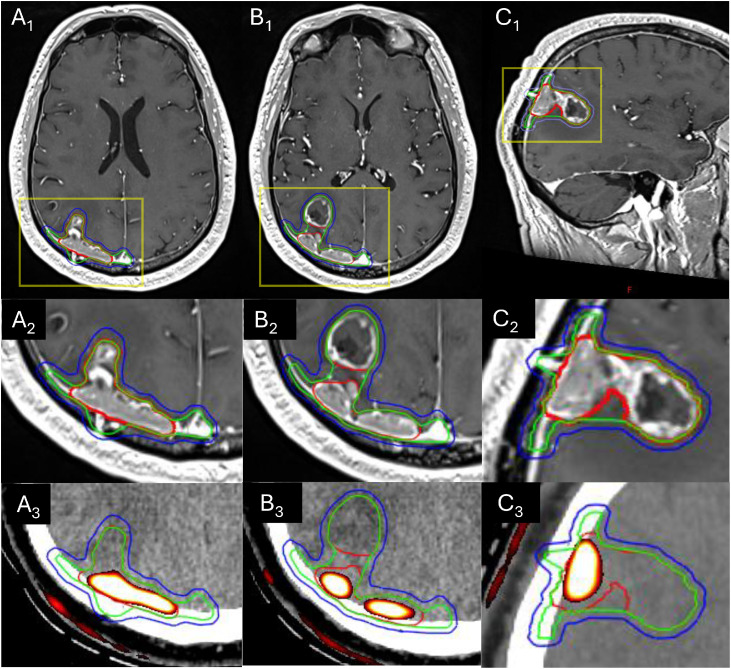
Preoperative reirradiation target volumes for the right parietal lesion with a magnetic resonance imaging (MRI) scan T1c+ and an ¹⁸F-fluciclovine positron emission tomography (PET) scan fusion. Gross tumor volume (GTV, red) encompasses the prior laser interstitial thermal therapy (LITT) tract and operative changes, contrast-enhancing regions, nodular dural disease on an MRI scan, and hypermetabolic regions on an ¹⁸F-fluciclovine PET scan. Clinical target volume (CTV, green) represents an anatomically tailored 3 to 5 mm expansion along the meninges with inclusion of the superior sagittal sinus. Planning target volume (PTV, blue) represents a 2 mm expansion from the CTV. Yellow boxes in A_1_, B_1_, and C_1_ denote magnified views in the middle and lower rows. (A_1_) Full axial MRI scan T1c+ fusion at the level of the burr hole and LITT tract, with a corresponding magnified MRI scan T1c+ view in (A_2_) and planning computed tomography (CT)/PET scan overlay in (A_3_). (B_1_) Full axial MRI scan T1c+ fusion at a more inferior level of the lesion, where the cavitary parenchymal component is more distinct from the nodular dural disease; corresponding magnified views of T1c+ and DSC perfusion overlays are shown in (B_2_) and (B_3_), respectively. (C_1_) Sagittal MRI scan T1c+ at the level of the burr hole and LITT tract, with enhancing parenchymal and nodular dural disease encompassed within the target volumes; corresponding magnified views of T1c+ and DSC perfusion overlays are shown in (C_2_) and (C_3_), respectively.
